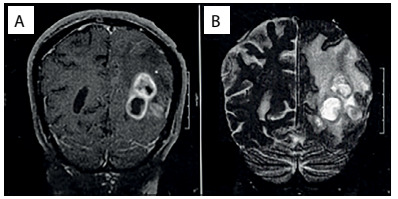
A) Coronal T1-weighted magnetic resonance imaging (MRI) showing enhanced left occipital lesions. B) Coronal T2 MRI showing extensive edema surrounding left occipital lesions.
